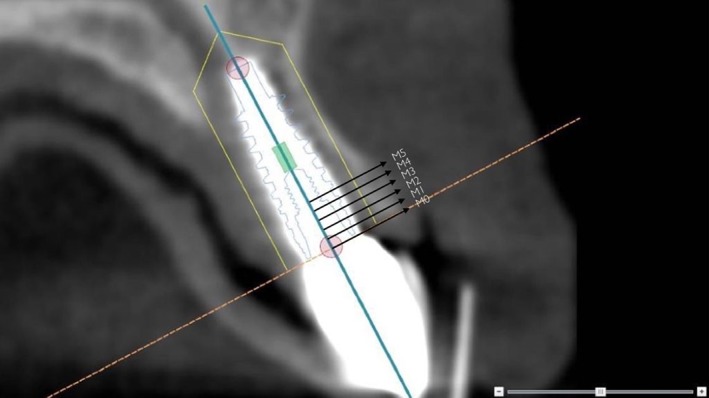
Measurement on a 1‐month cone‐beam computed tomography from the central axis of a planning implant, placed in the position of the actual implant, to the outer surface of the buccal bony wall at the implant with the planning program NobelClinician
